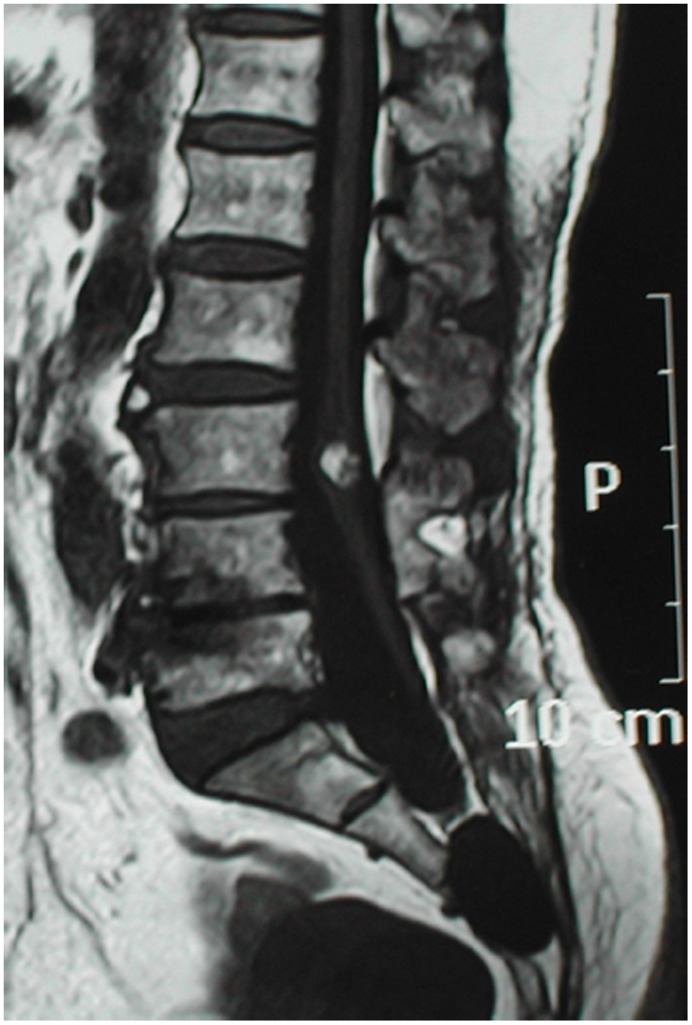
MRI findings of a cyst in the sacral spinal canal, a low-lying tethered cord and a intramedullary tumor at L3 level.
